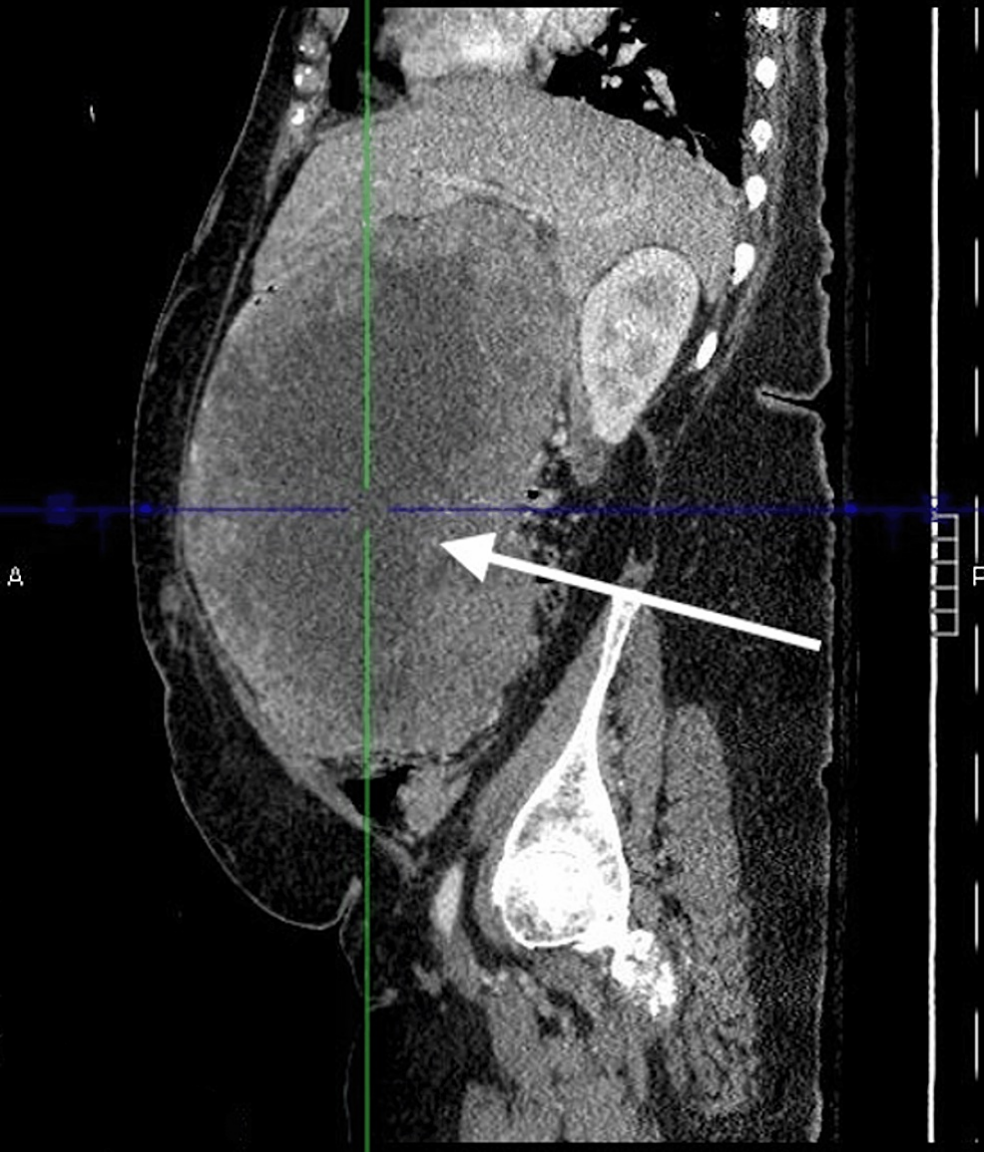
CT abdomen and pelvis CT Abdomen/pelvis sagittal plane with the white arrow pointing to a large intraperitoneal mass with central necrosis and peripheral enhancement
